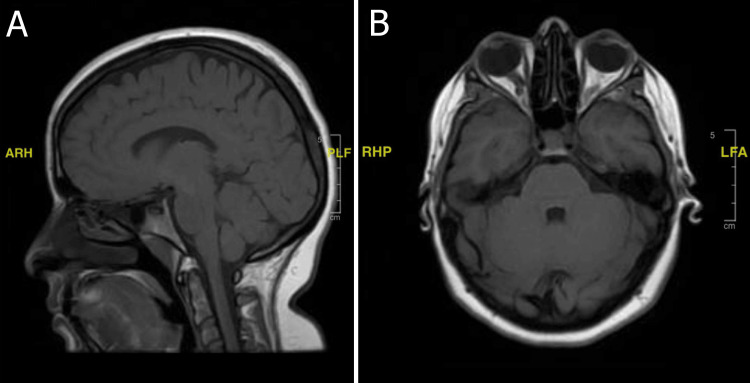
(A) A FLAIR sequence MRI of the brain without contrast in sagittal view and (B) a FLAIR sequence MRI of the brain without contrast in axial view, both showing the bilateral tortuosity of the intraconal optic nerve with the prominence of the perioptic CSF spaces in conjunction with optic nerve papillae protruding into the vitreous space of the globe, relating to papilledema. FLAIR, fluid-attenuated inversion recovery; MRI, magnetic resonance imaging; CSF, cerebrospinal fluid; ARH, anterior, right, head; PLF, posterior, left, foot; RHP, right, head, posterior; LFA, left, foot, anterior
